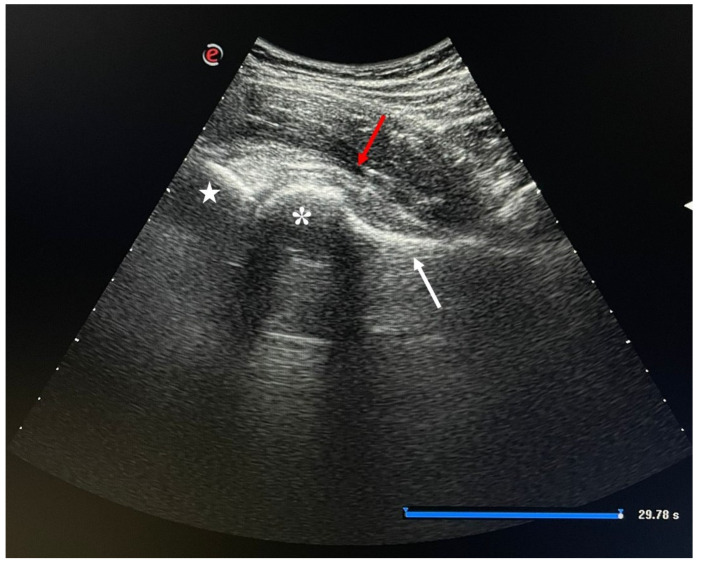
Ultrasound long-axis view of the hip as it is visualized during injection. Star = anterior rim of acetabulum; * = femoral head; white arrow = femoral neck; red arrow = joint capsule.
